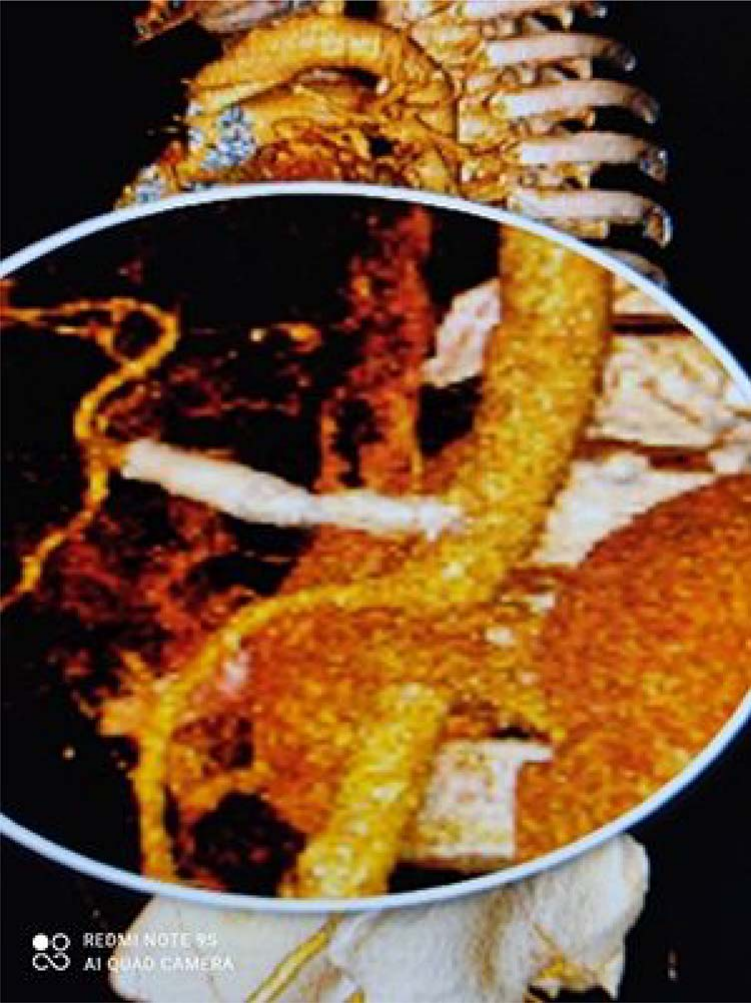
3D dimensional CT angiography shows CAA after intervention.
